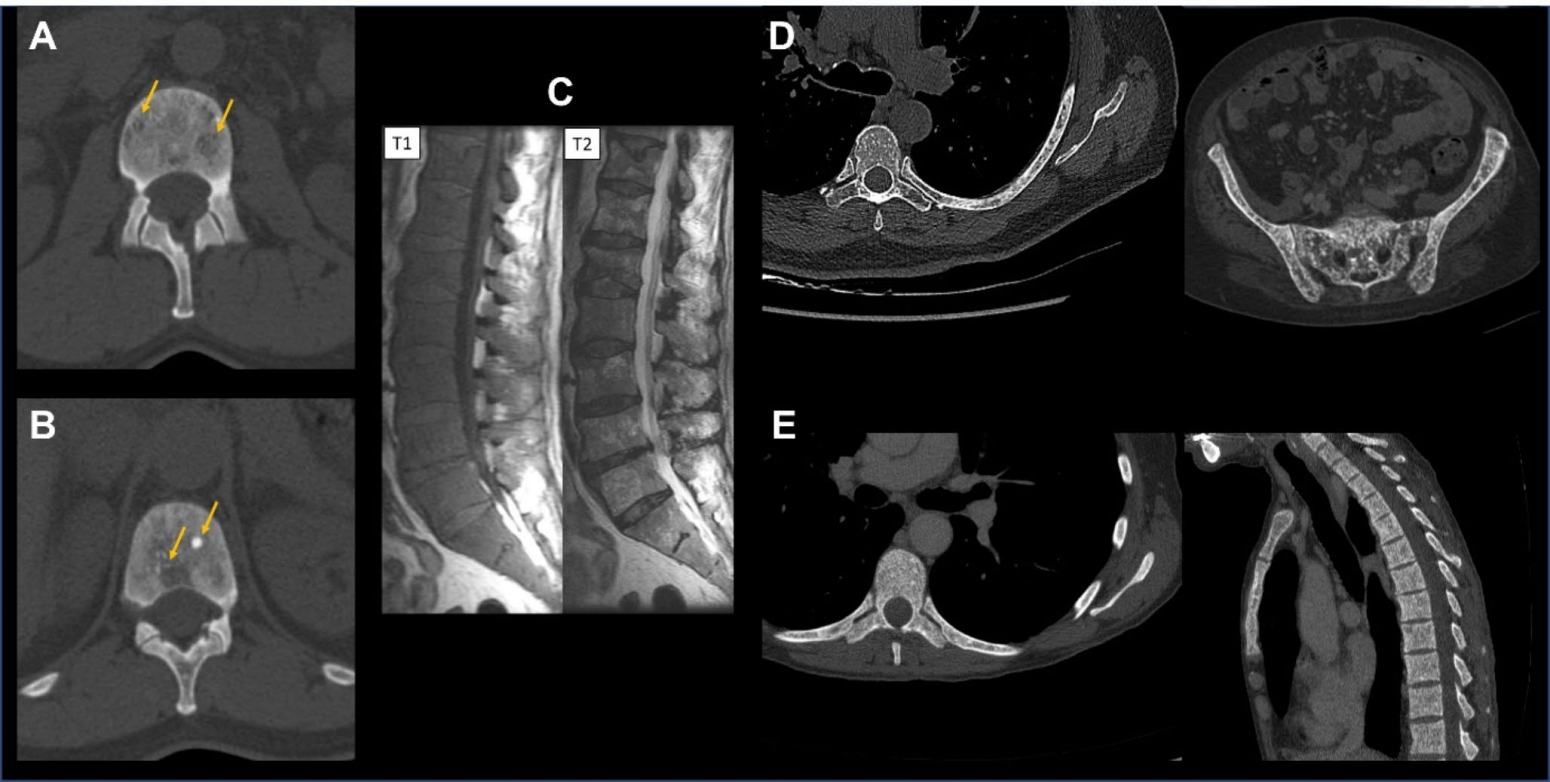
Main bone radiological involvement in systemic mastocytosis. (A) Spine CT-scan with vertebral focal osteolytic lesions. (B) Spine CT-scan with mixed lesions on the same vertebra with both focal osteolysis and focal osteocondensation. (C) Spine MRI with diffuse infiltration consisting of hypointense signal in T1-weighted sequence and heterogeneous T2 signal in fat-saturated sequence. (D) Spine and pelvis CT-scan with disseminated punctiform osteocondensations. (E) Spine CT-scan with diffuse bone marrow sclerosis/osteosclerosis
